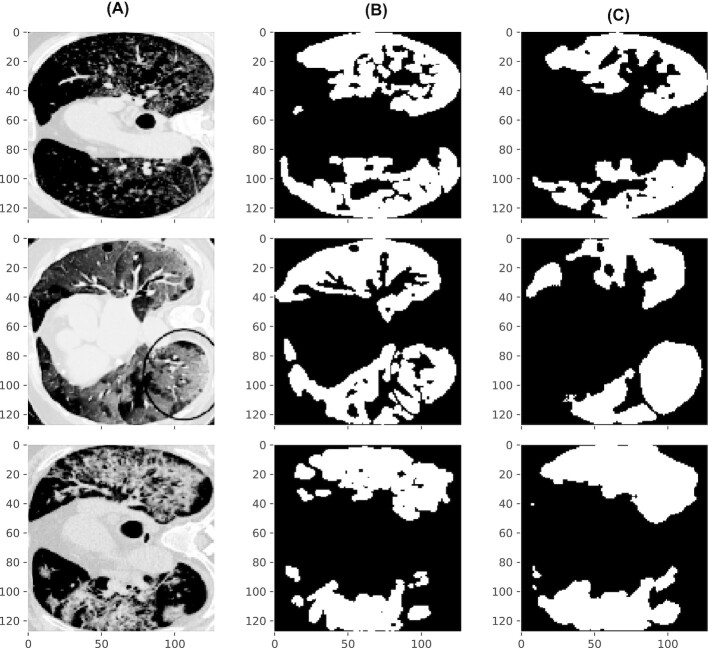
Original CT scan images (A), corresponding ground-truth masks of original CT scan images (B), and the predicted masks (C). Masks are COVID-19 infected regions in the corresponding CT scan images. The ground-truth and predicted masks show high similarity [44].
